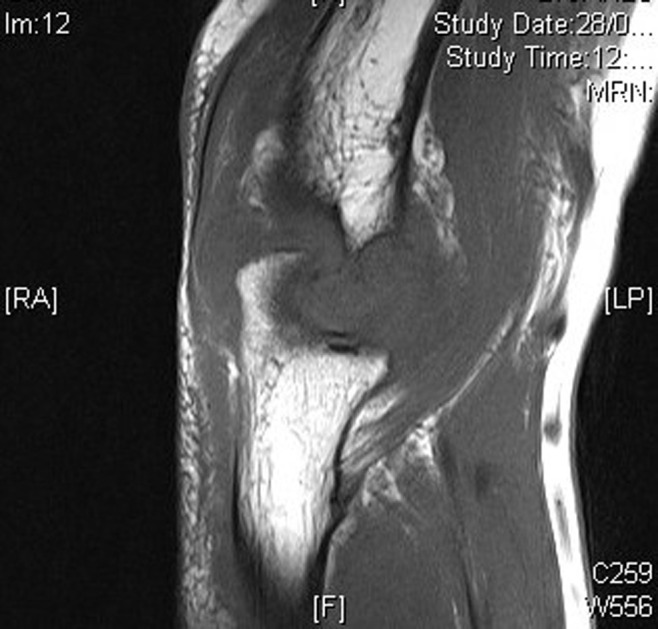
magnetic resonance imaging (MRI) showing the destructive lesion of the bone cortex of the elbow junction
